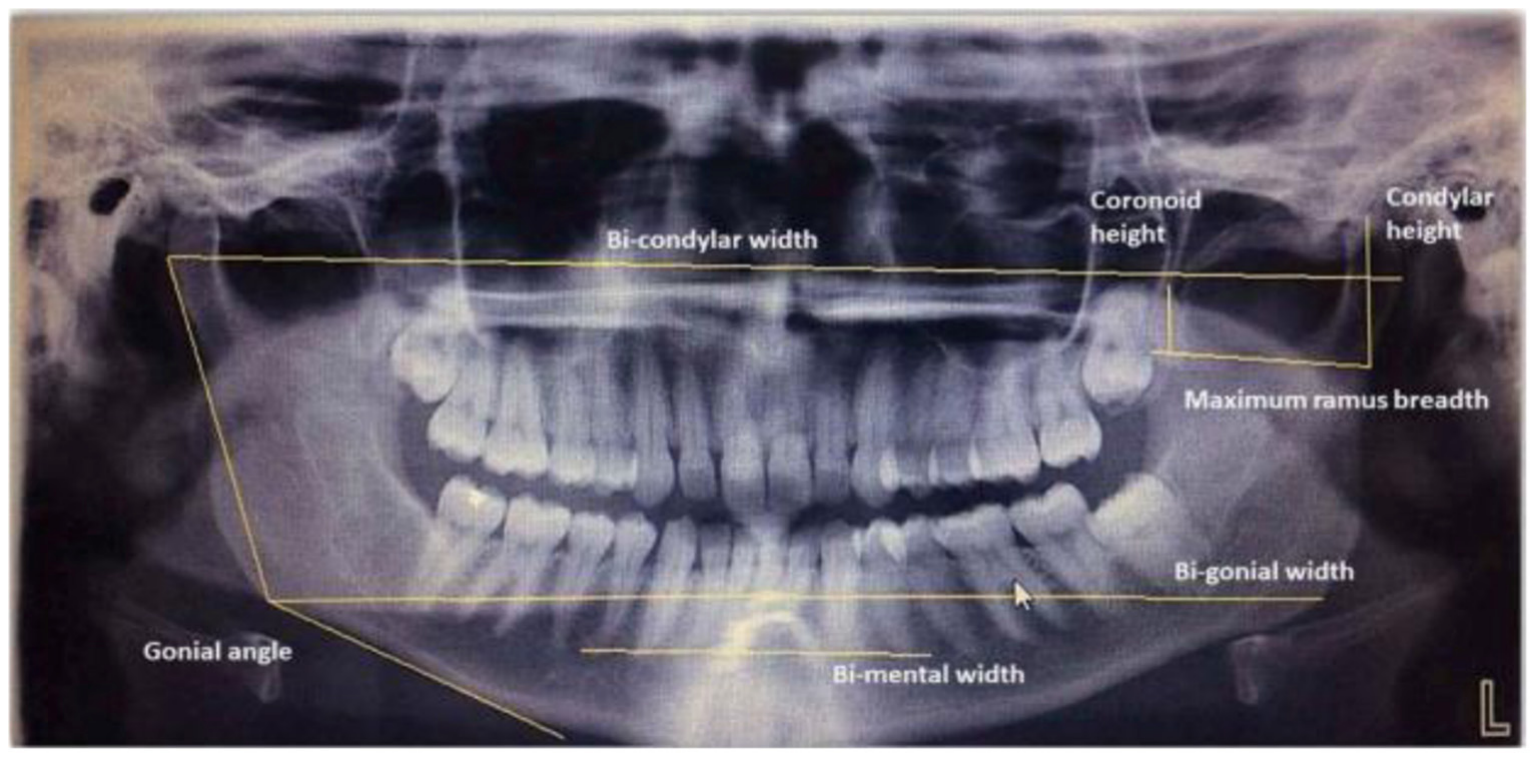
Morphometric parameter measurements done on digital panoramic radiographs by Raj and Ramesh (2013), Kumar et al. (2016), and More et al. (2017).
